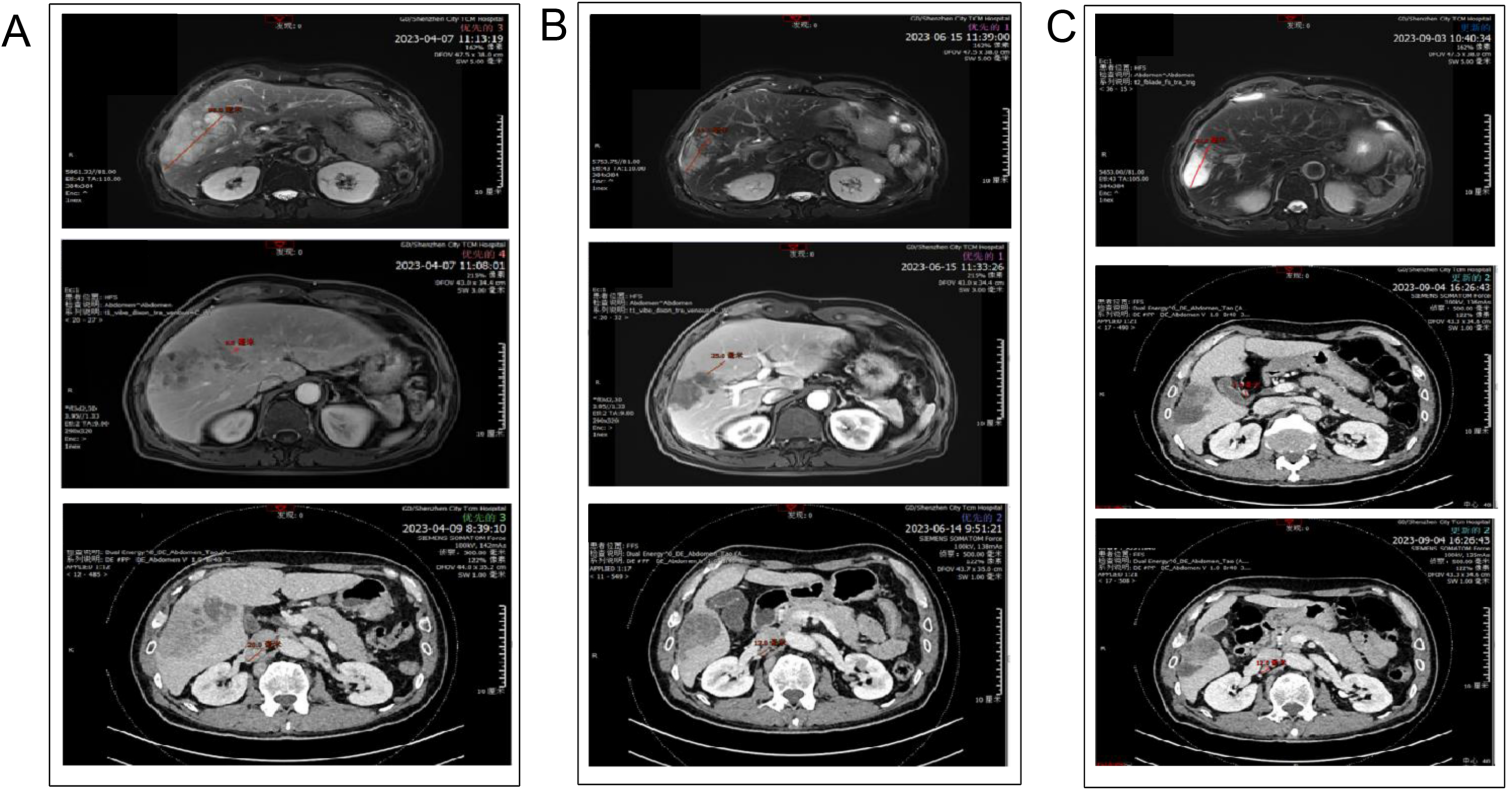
Computed tomography (CT) scans. (A) CT scan of the patient in April 2023. (B) CT scan of the patient in June 2023. (C) CT scan of the patient in September 2023.
